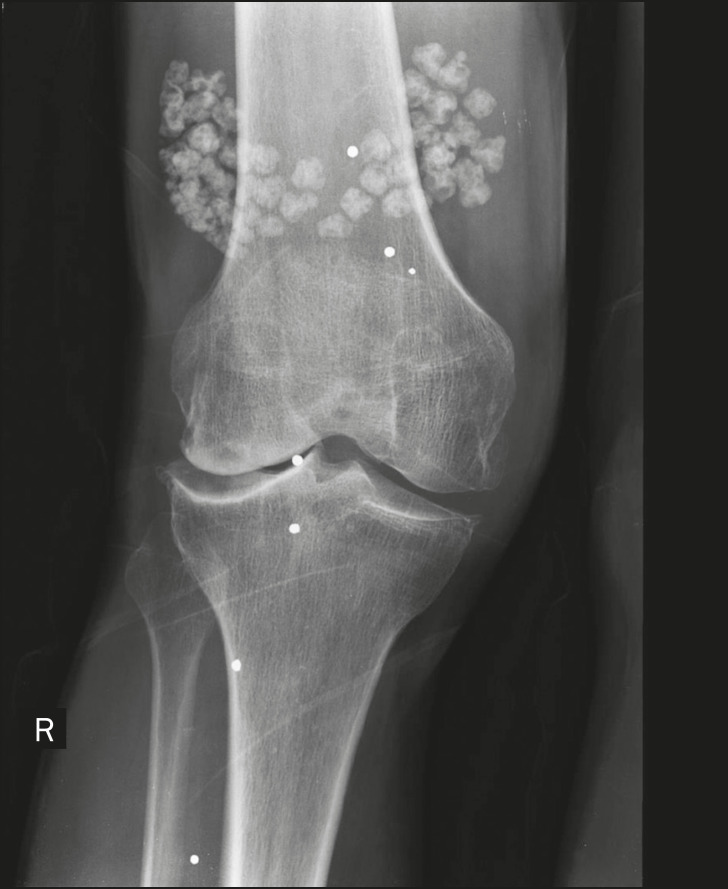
Synovial osteochondromatosis. Frontal X-ray of the knee. The radiographic appearance is often diagnostic, showing numerous rounded calcified bodies within the joint space, usually measuring 1–20 mm in diameter, accompanied by joint effusion.
